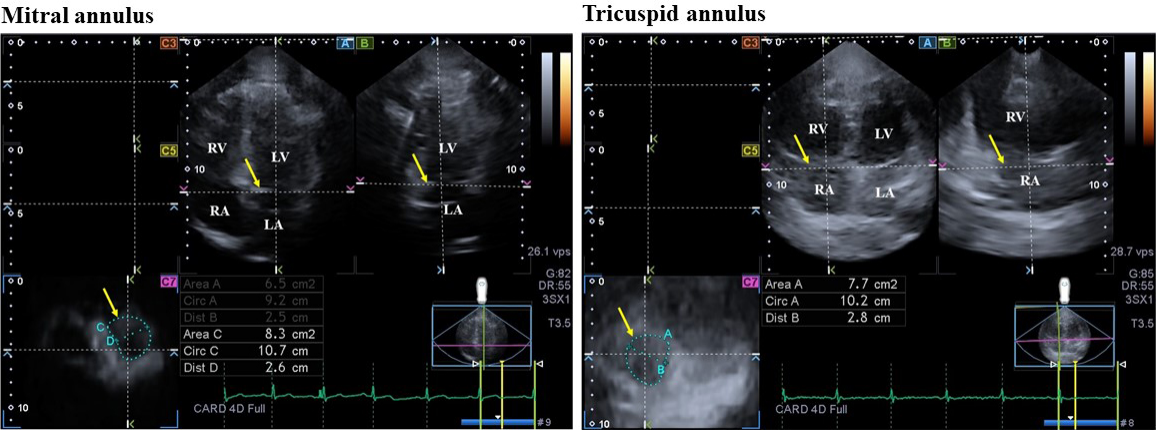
Three-dimensional (3D) echocardiographic assessment of mitral
